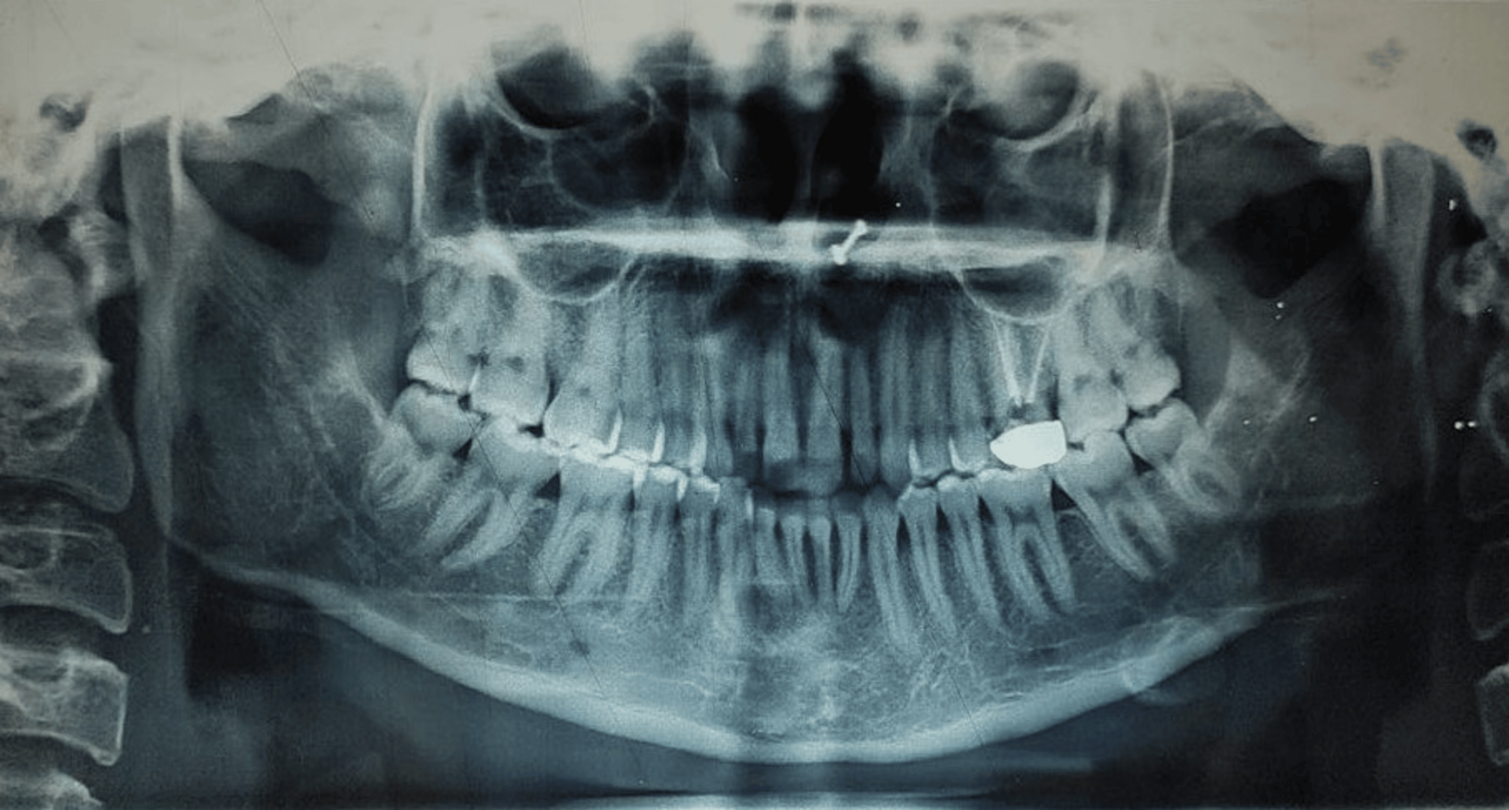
Pretreatment orthopantomogram.
